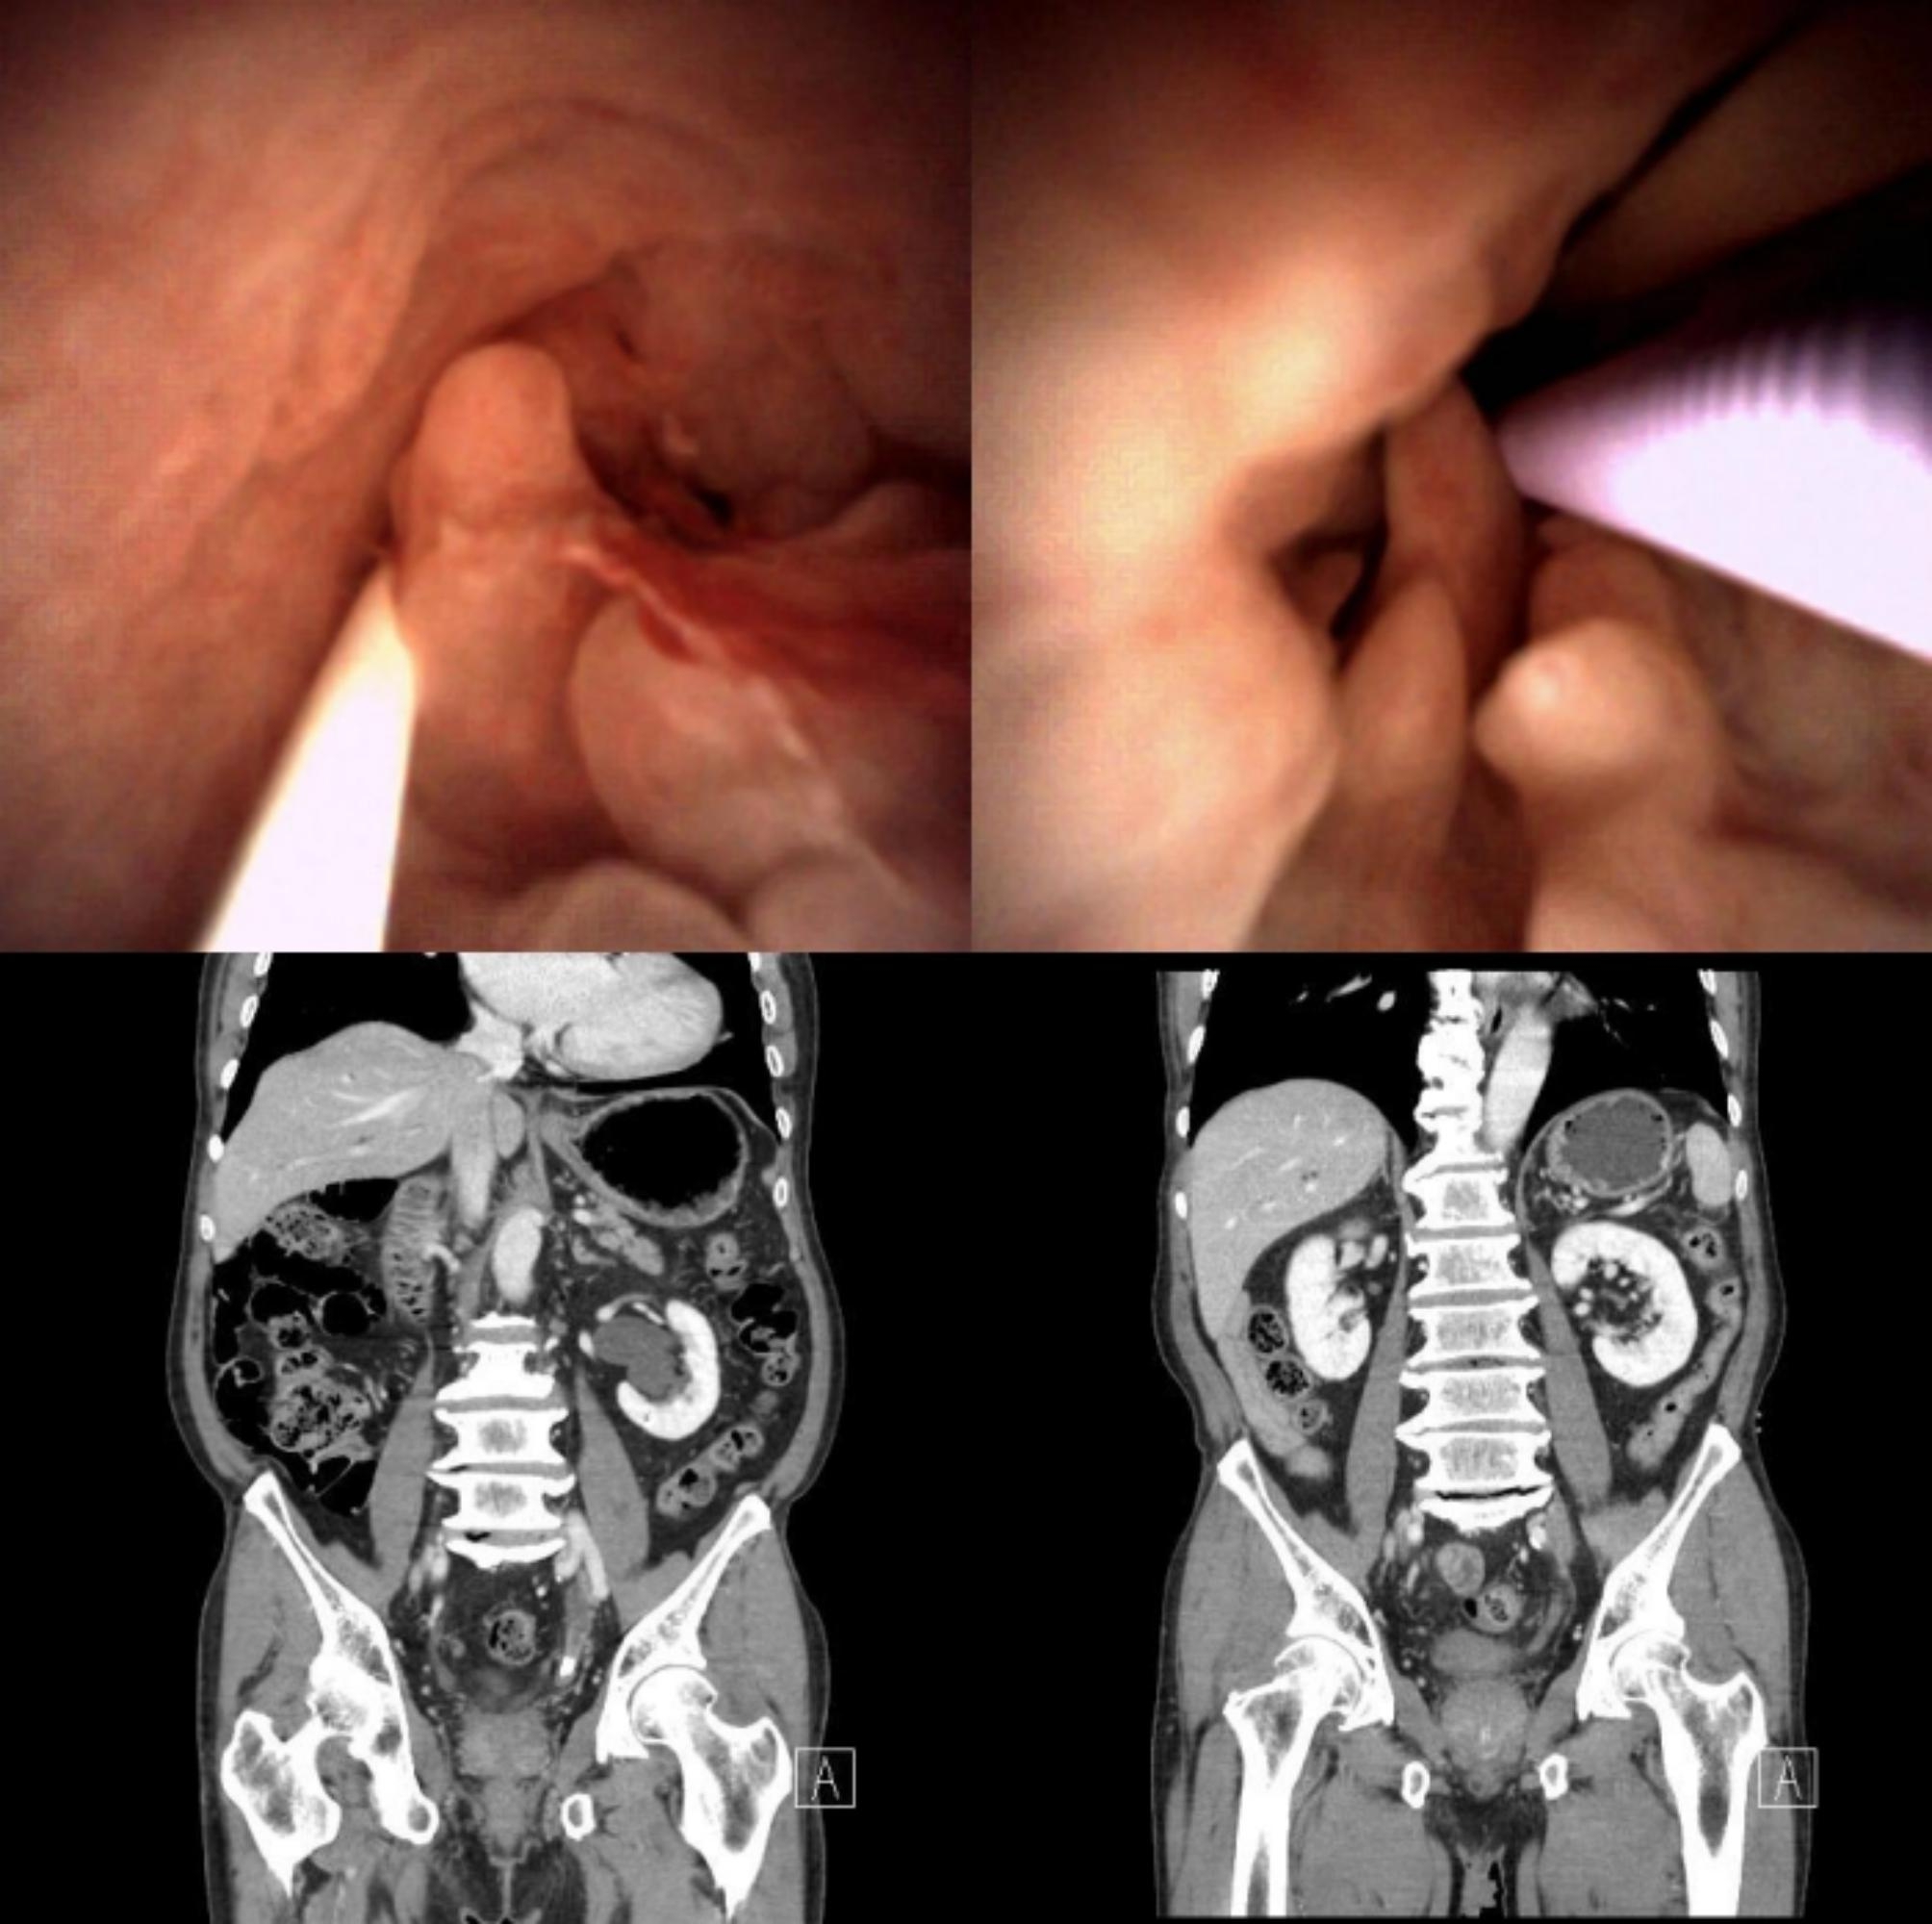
Fibroepithelial polyps with ureter stone Fibroepithelial polyp during ureter stone treatment (left top). Lt. lower ureter stone in preoperative computed tomography (left bottom). Persistent fibroepithelial polyp during follow-up ureteroscopy (Right top). No more hydronephrosis in postoperative follow-up computed tomography (Right bottom)
